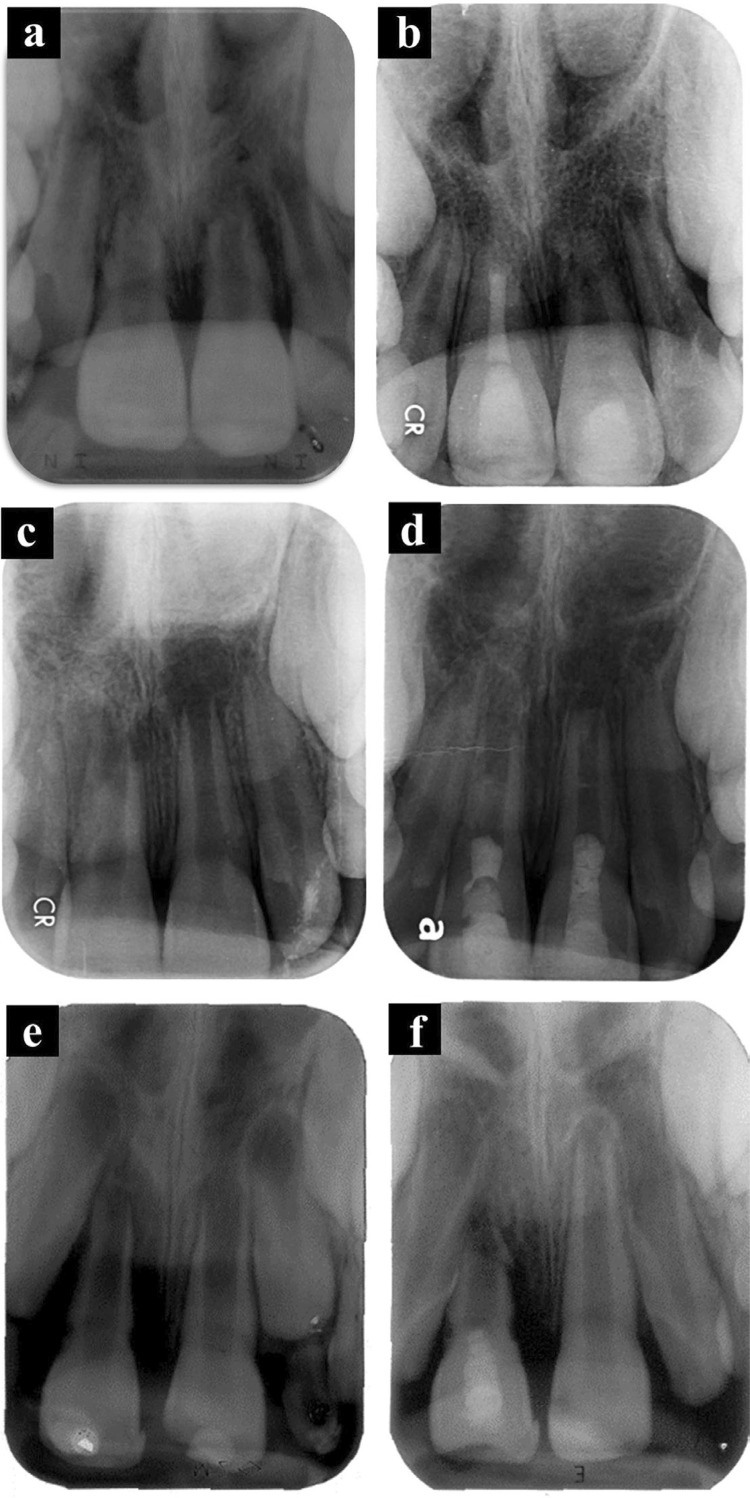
(a) Initial radiography of a 9-year old girl with extrusive luxation of the maxillary left central incisor after a fall; (b) After 24 months, showing continued root development. (c) Initial radiography of a 9-year old boy with extrusive luxation of the right and left maxillary central incisors after sports activity. This radiography showed signs of root resorption. This patient took 3 years to seek treatment in the dental trauma service, after receiving initial care in a hospital; (d) After 18 months, showing periapical healing, incomplete apical closure, absence of a significant increase in root length and root width, and stabilization of root resorption. (e) Preoperative radiograph. (f) Follow-up radiograph 9 months after treatment showing replacement resorption. Subsequently, the patient retraumatized the tooth, and it was avulsed
